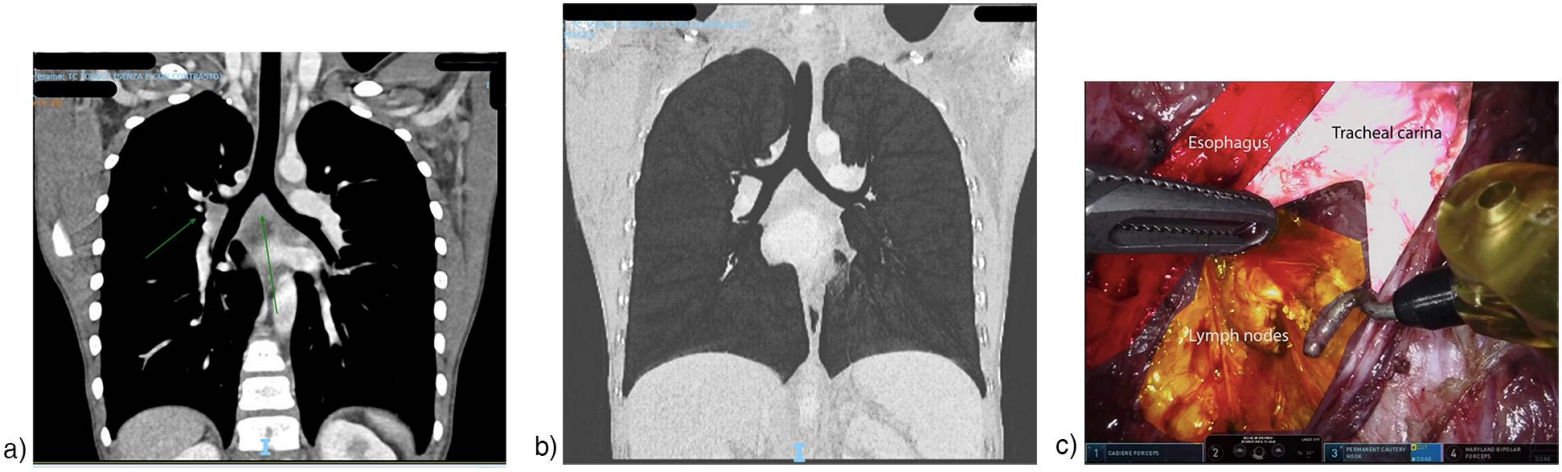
(a,b) preoperative computed tomography (CT) images showing the localization of the lymph nodes selected for biopsy. (c) Intraoperative image documenting the surgical dissection of the lymph nodes.
